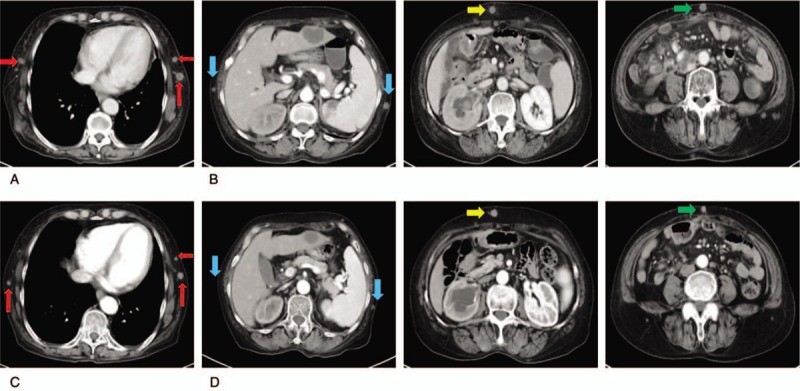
CT indicating cutaneous lesions on the chest wall (A) and abdomen wall (B). (C) Chest CT and abdominal CT (D) after 2 cycles of FOLFIRI and cetuximab treatment. CT = computed tomography. FOLFIRI = irinotecan, leucovorin, and fluorouracil.
